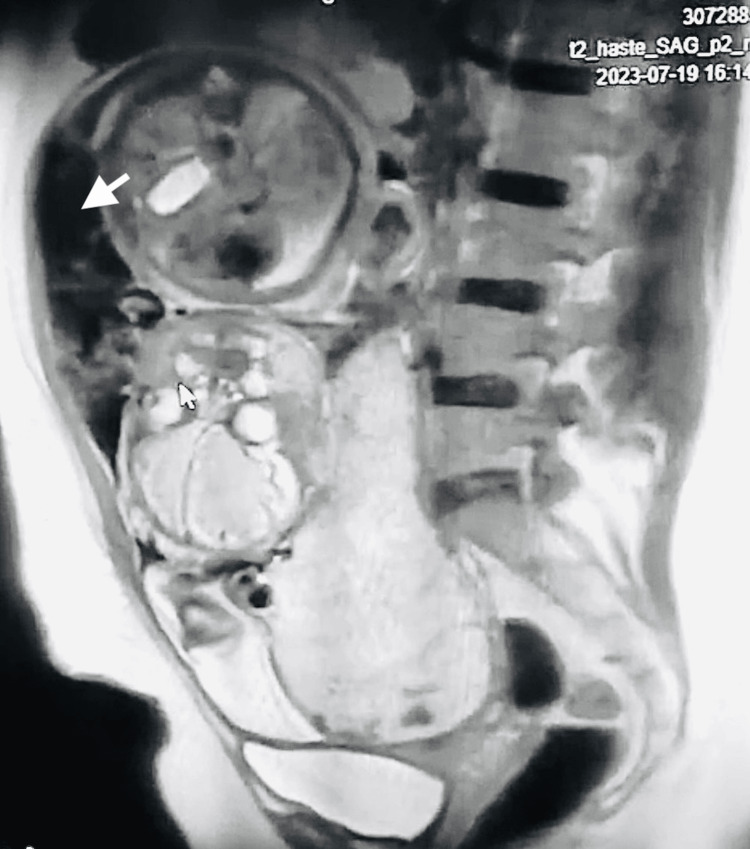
MRI showing placenta accrete with large white arrow (Case 2).
